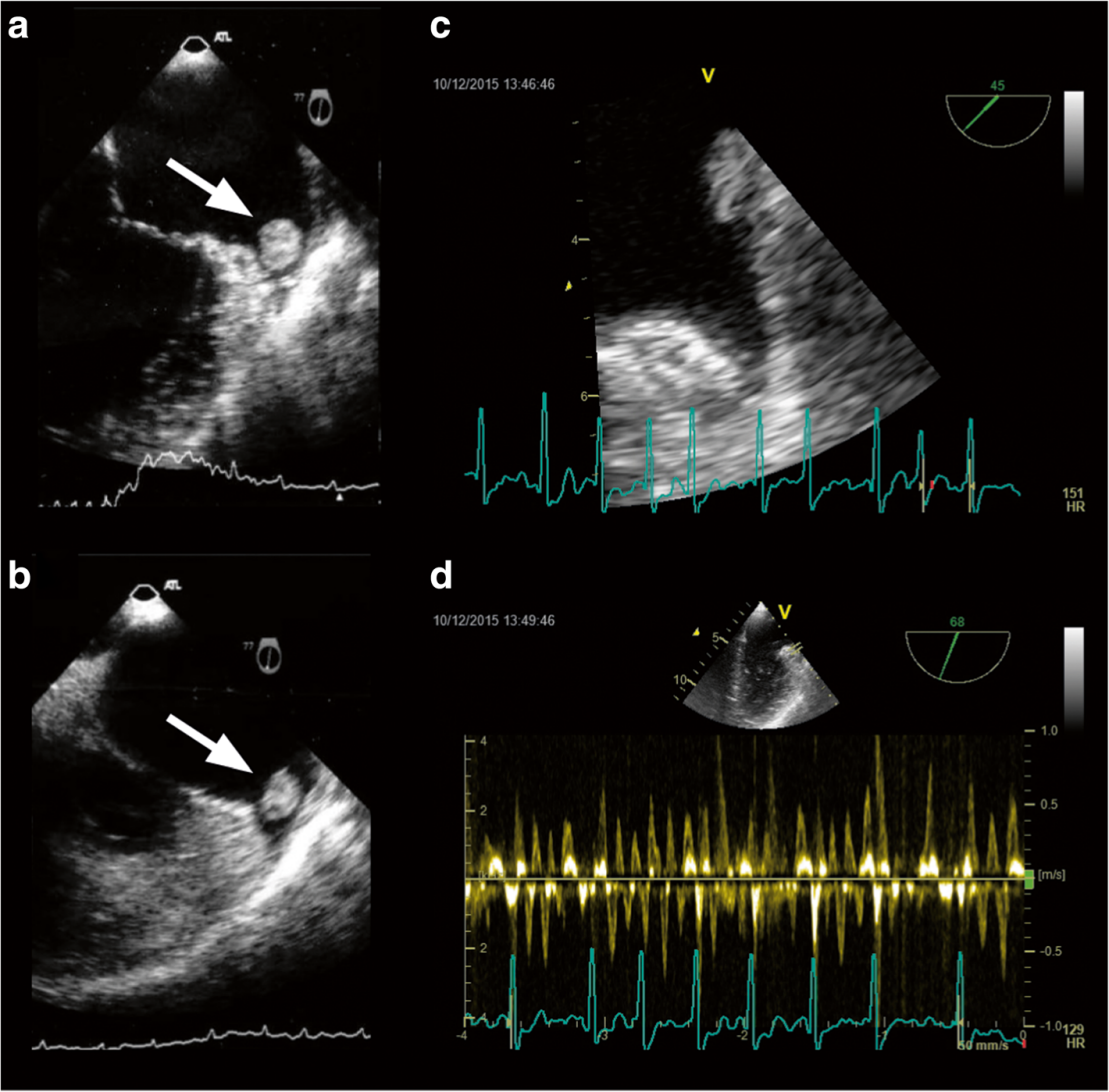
a Transesophageal echocardiography at diagnosis showing a mass in the left atrial appendage. b Transesophageal echocardiography after 7 days of treatment with intravenous unfractionated heparin showing a mass in the left atrial appendage. c Transesophageal echocardiography after 3 months of rivaroxaban use showing no visible mass in the left atrial appendage. d Doppler with high-speed blood flow in the appendage after 3 months of rivaroxaban use
